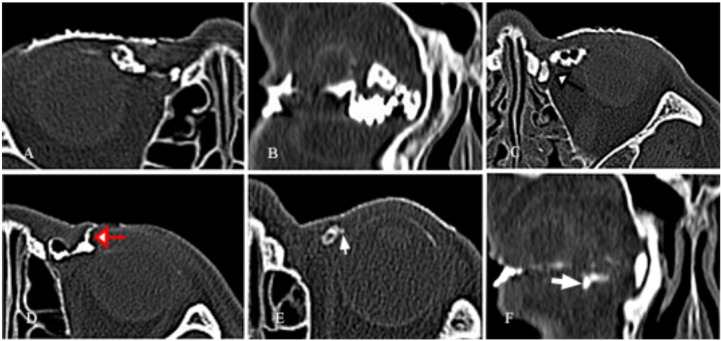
CT-DCG imaging features of primary canaliculitis.(A) Canalicular dilation towards the temporal side with both types of filling defects. (B) Dilations involved the entire length of the upper and lower canaliculi with both types of filling defects. (C) Dilation and filling defects of the canaliculus accompanied by dilation of the lacrimal sac (black arrow). (D) A skin fistula connected to the affected inferior canaliculus (red arrow). (E) The diverticulum formed on the temporal side of the canaliculus (thin white arrow). (F) The diverticulum formed below the vertical segment of the lower canaliculus (thick white arrow).
